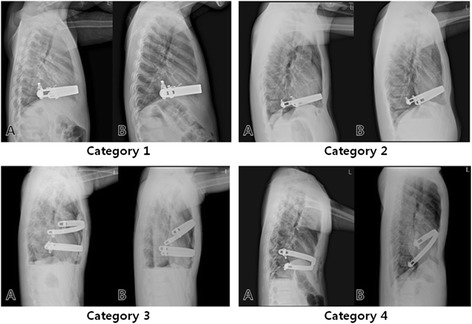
Radiographs describing the four categories of bar displacement. a preoperative; b: postoperative
